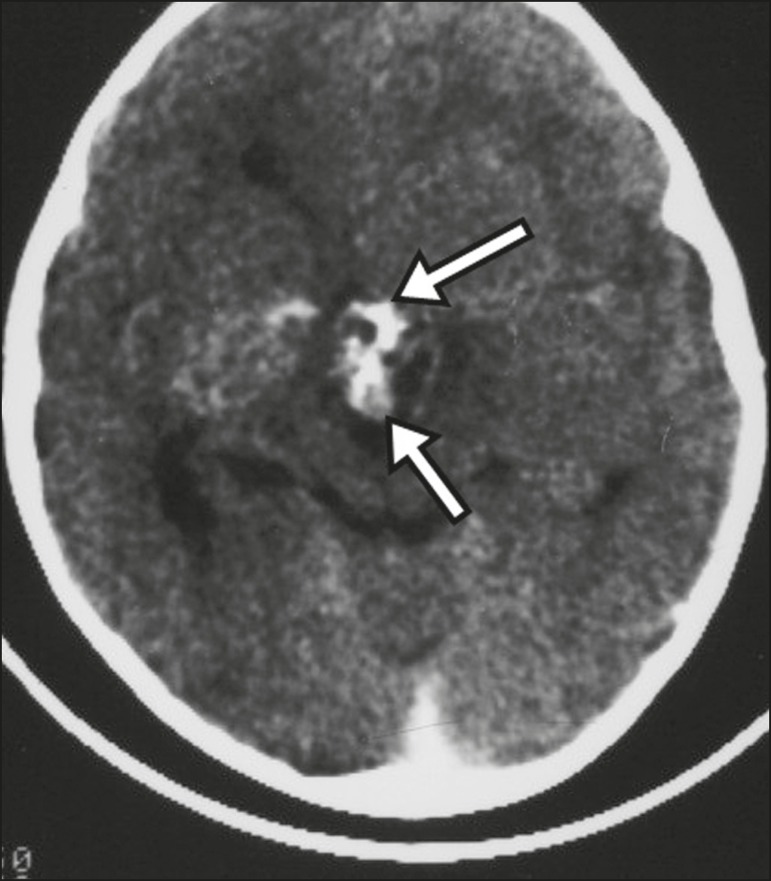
Craniopharyngioma. Non-contrast-enhanced CT scan showing calcifications
